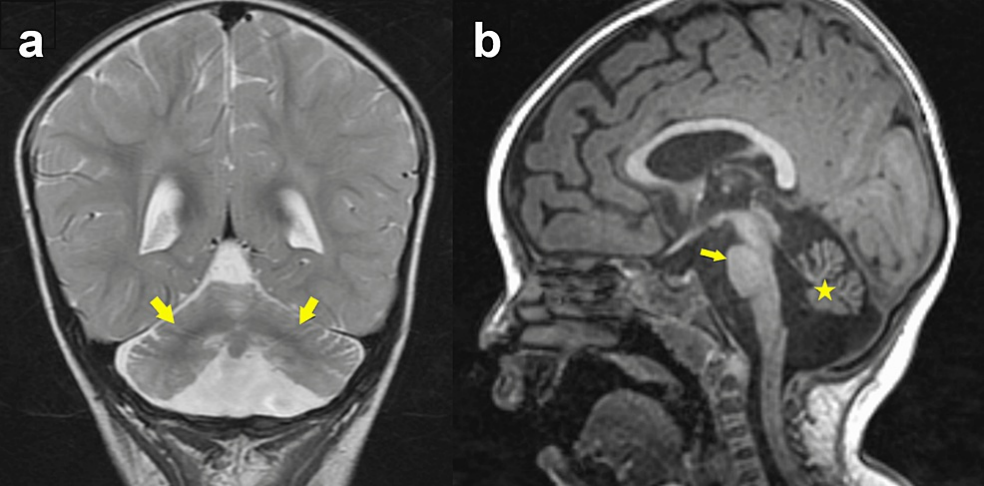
MRI findings in the older sibling. Coronal T2 MRI image (a) demonstrates the splayed hypoplastic appearing bilateral cerebellar hemispheres (arrows). The cerebellum appears to be “floating” in the posterior fossa. Sagittal T1 MRI image in the midline (b) demonstrates a small pons (arrow) and hypoplastic cerebellum (star)
